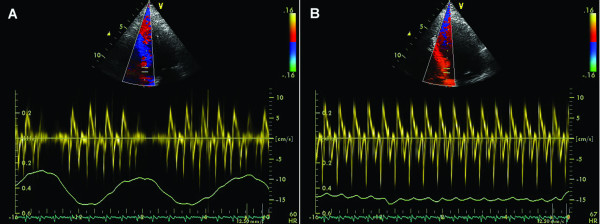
Septal annular velocities measured by tissue Doppler echocardiography during quiet respiration (A), and during end-expiratory apnea (B).
